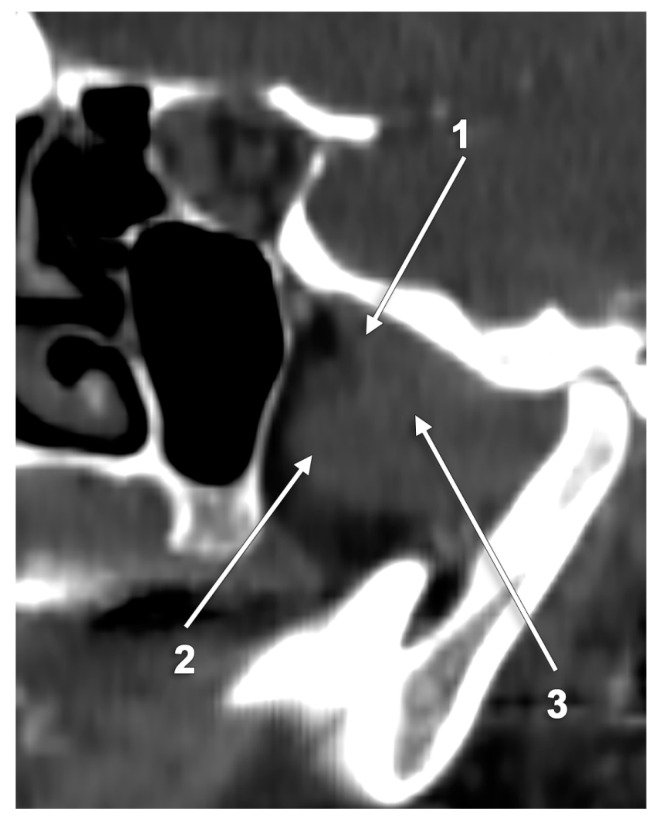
Sagittal CT slice through the lateral pterygoid muscle: 1. superior head; 2. inferior head; and 3. merged heads.
